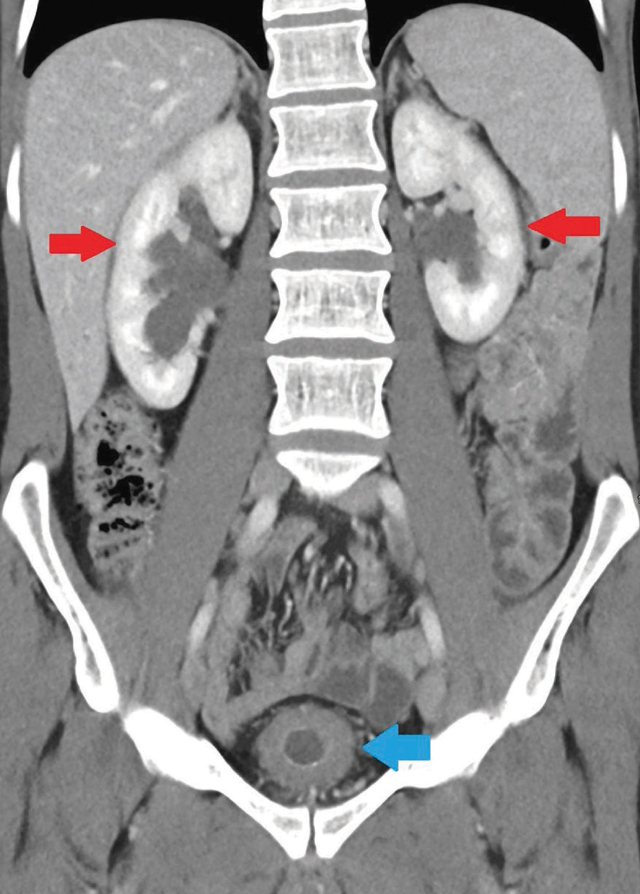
A 26-year-old patient. Contrast-enhanced CT showing the shrunken bladder with thickened wall (blue arrow) associated with bilateral hydronephrosis (red arrows).
